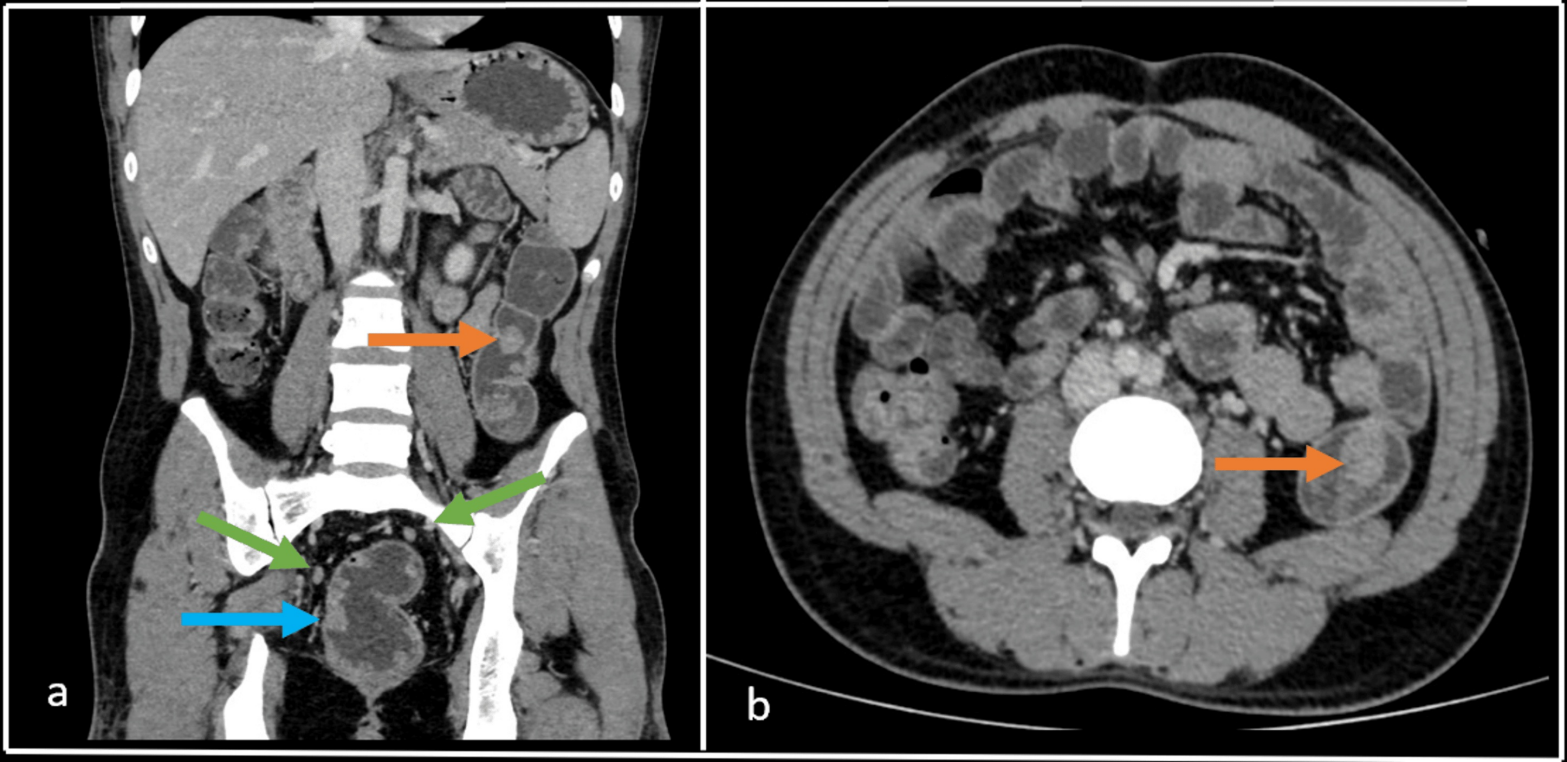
Contrast-enhanced CT abdomen coronal (a) and axial (b) venous phase shows the largest of the polyps in the rectum (blue arrow) and descending colon (orange arrow) measuring ~ 22 x 22 mm and 18 x 18 mm, respectively. Few homogenously enhancing sub-centimetric nodes were seen in mesorectum nodes (green arrow).
